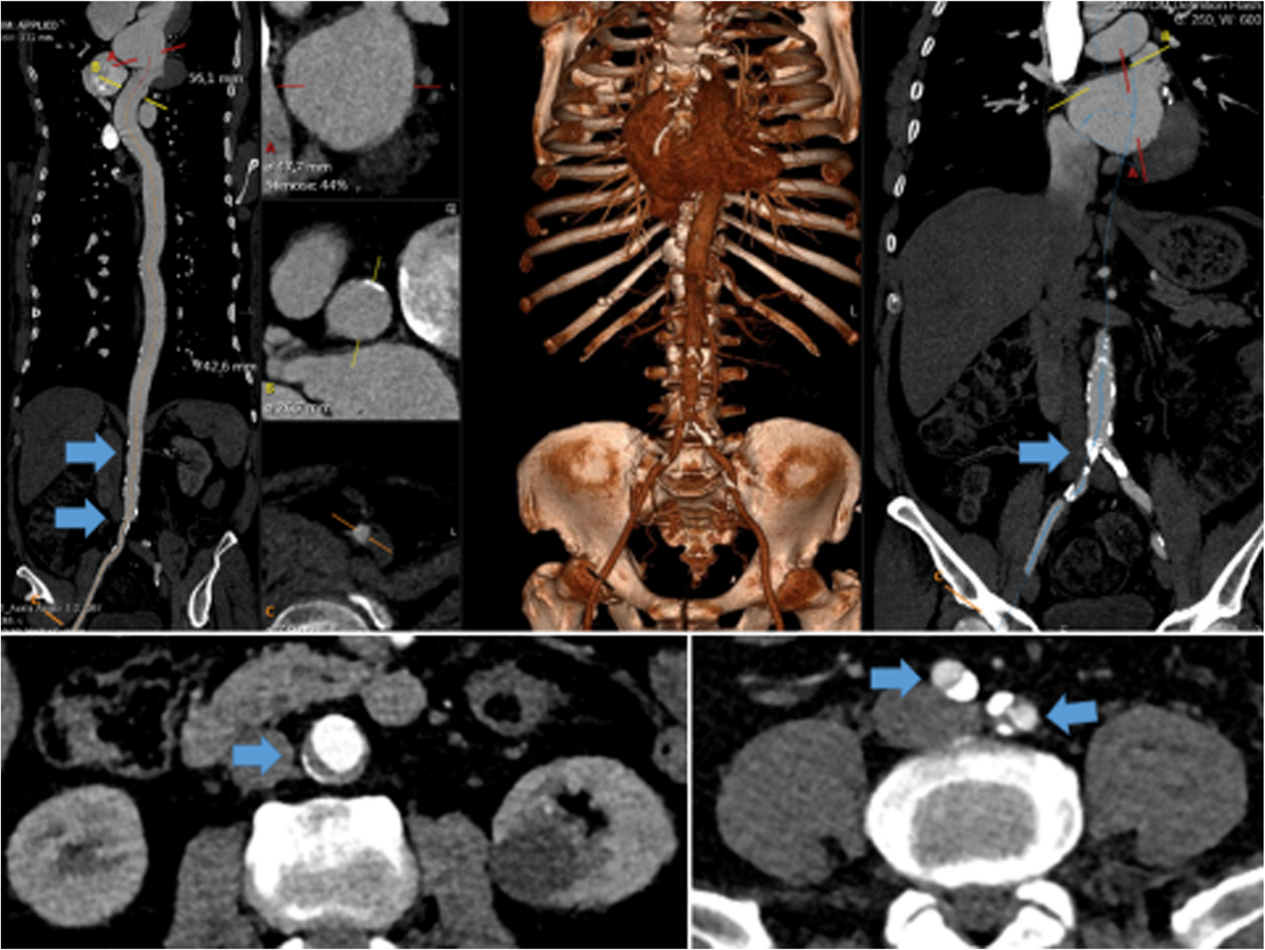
Revealed vascular pathologies in the performed preoperative advanced CT-A scans
